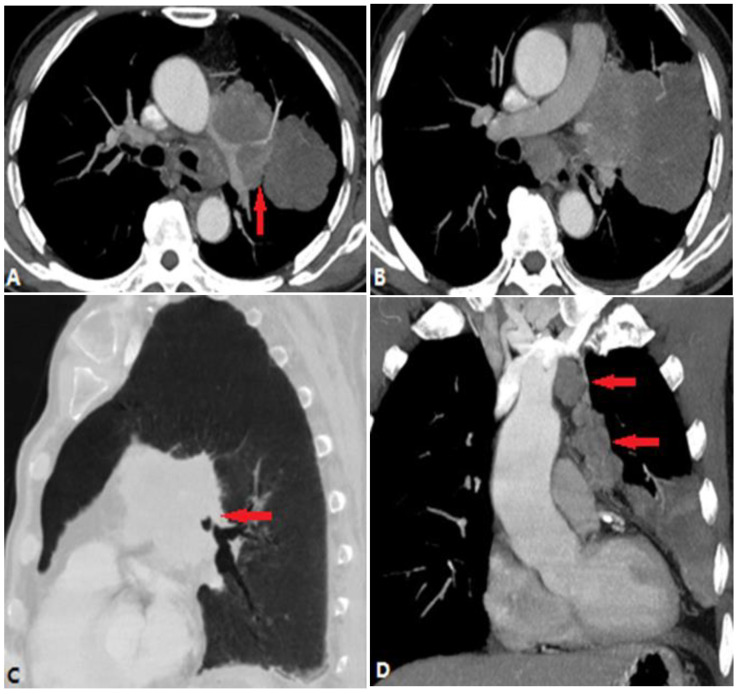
Tumor in a 50-year-old male with lung cancer. (A,B): Axial MIP shows that the tumor infiltrates and compresses the pulmonary arteries of the left lung, following the lumen narrowing (arrow). (C): Sagittal view shows the occluded bronchus (arrow). (D): Enlarged lymph nodes (arrows) on coronal CT due to metastasis. MIP—maximum-intensity projection.
